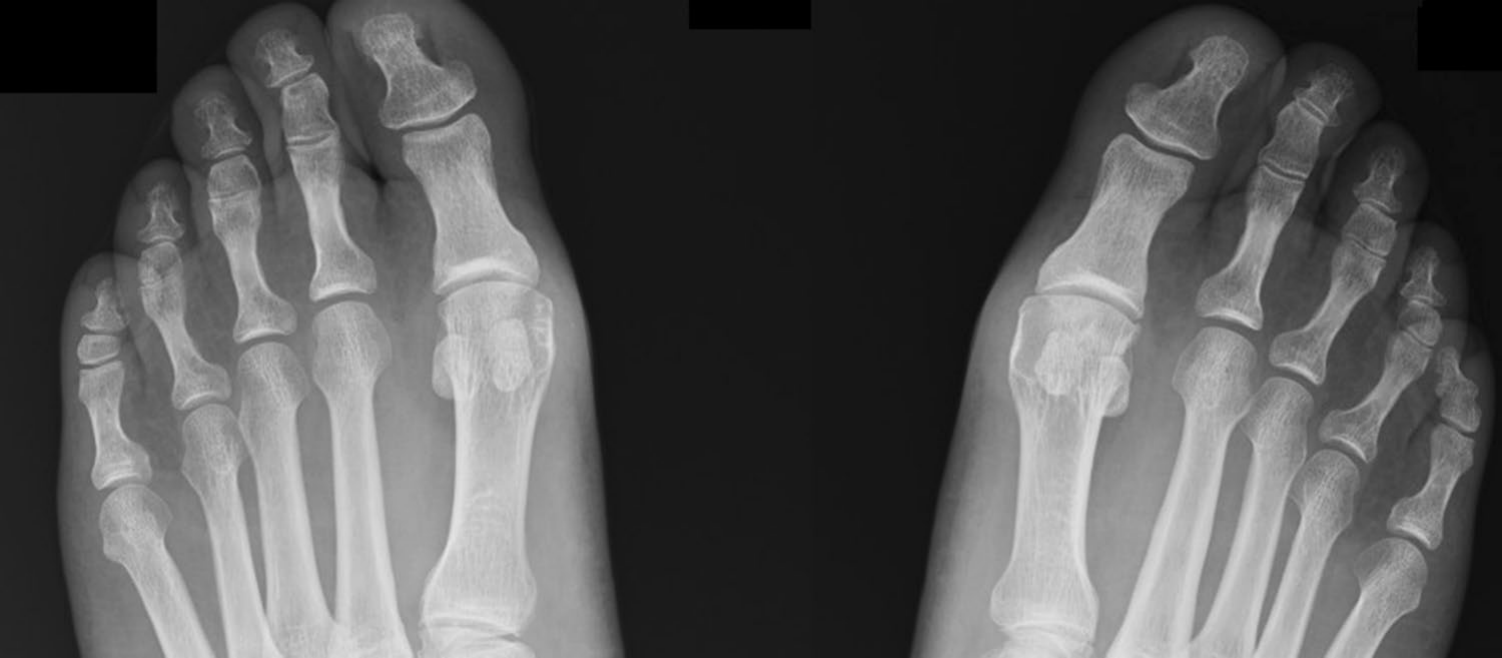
Bilateral dorso-planar foot radiograph of an adult shows a triphalangeal left 5th toe, and a biphalangeal right 5th toe
